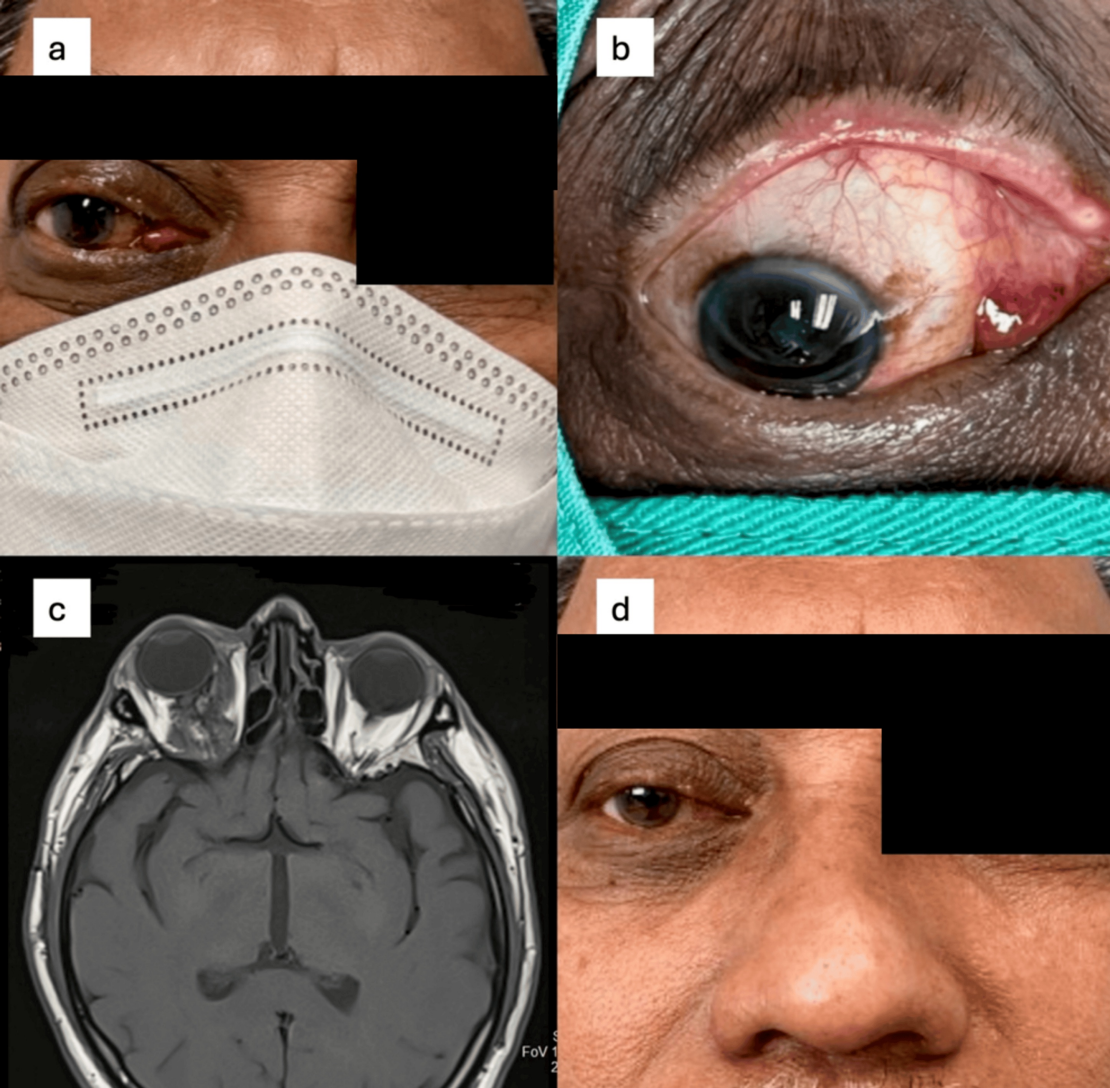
Case 3 on presentation showing right eye exotropia, proptosis, and conjunctiva chemosis medially (a). The eye was also displaced downward and laterally (b). MRI T1 axial showing a heterogenous extraconal/intraconal mass (c). Three months post the second intralesional bleomycin, the patient showed significant regression of the proptosis and conjunctival component (d).
